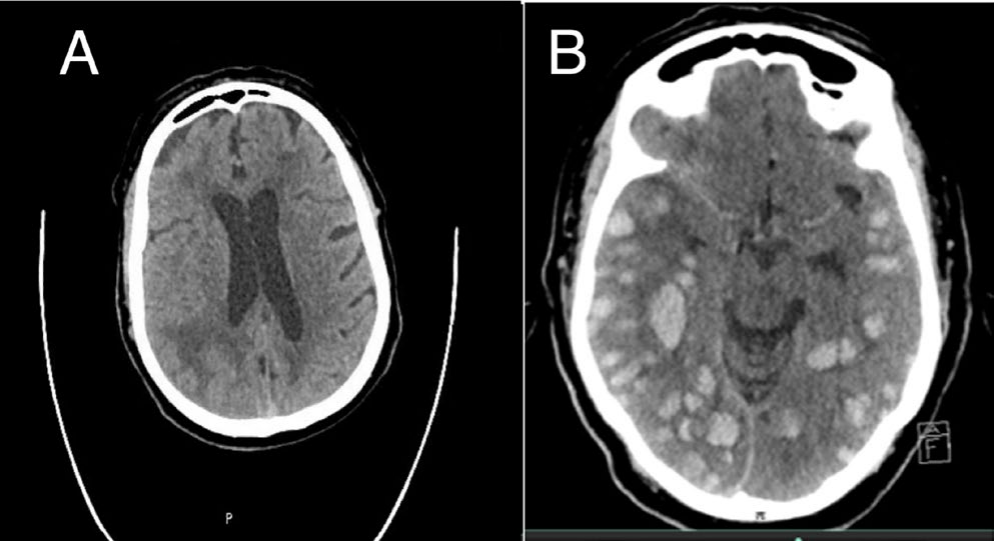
(A) Head CT scan showing hypo‐density in the right temporoparietal area. (B) Post‐thrombolysis head CT scan showing multifocal diffuse intracranial hemorrhage.
